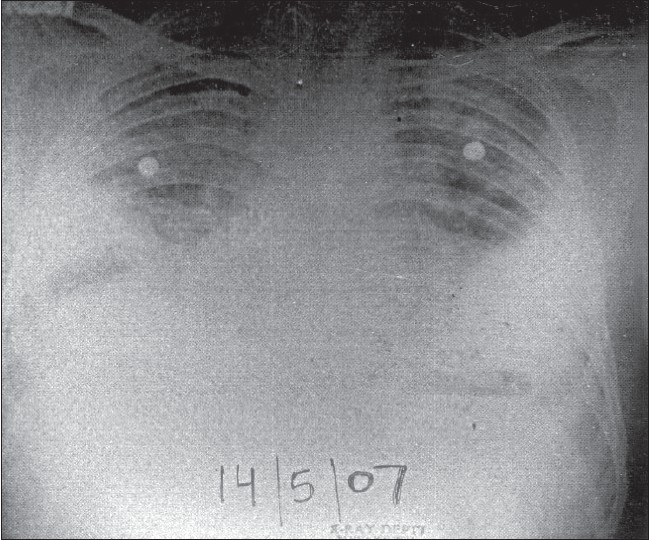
Chest radiograph done three days prior to hospitalization showing nonhomogeneous opacity in right lower zone with ill-defined margins, suggestive of a parenchymal consolidation process, and left-sided pleural effusion
